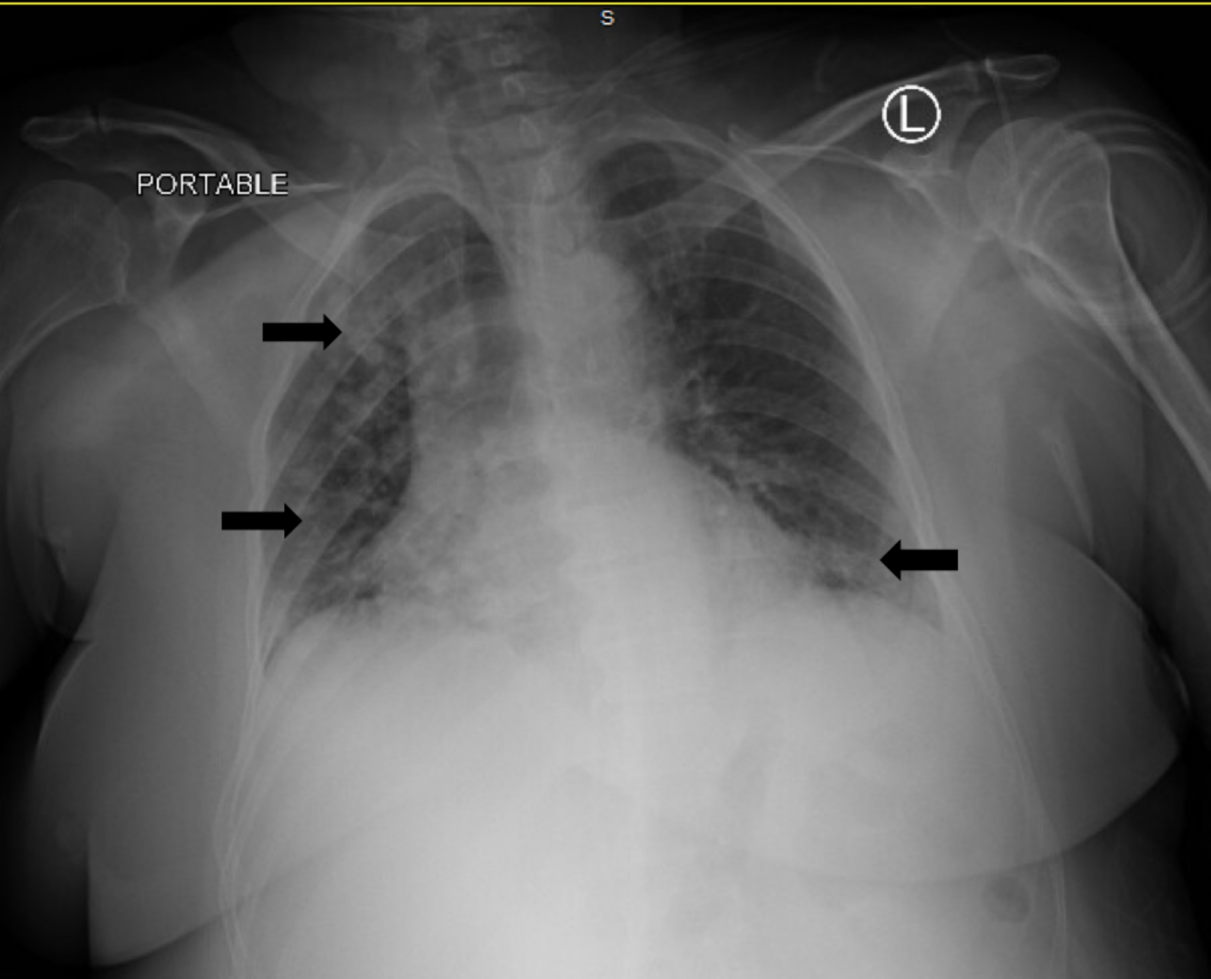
Chest X-ray reveals bilateral infiltration
